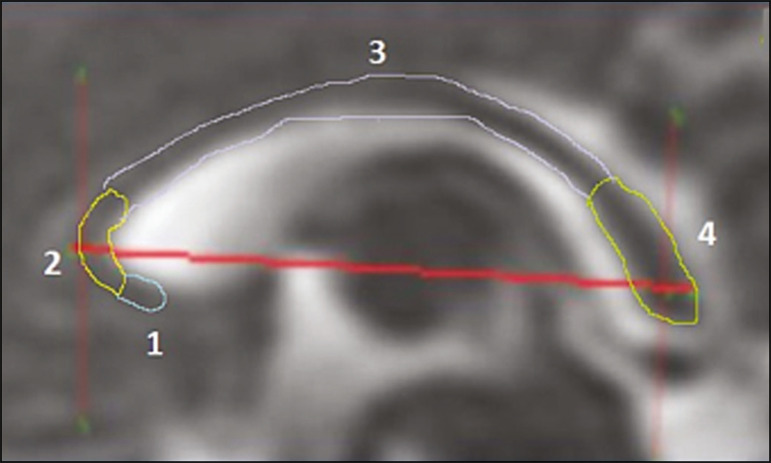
MRI of the corpus callosum. The red line represents the measurement of its length. 1, rostrum; 2, knee; 3, body; 4, splenium.
